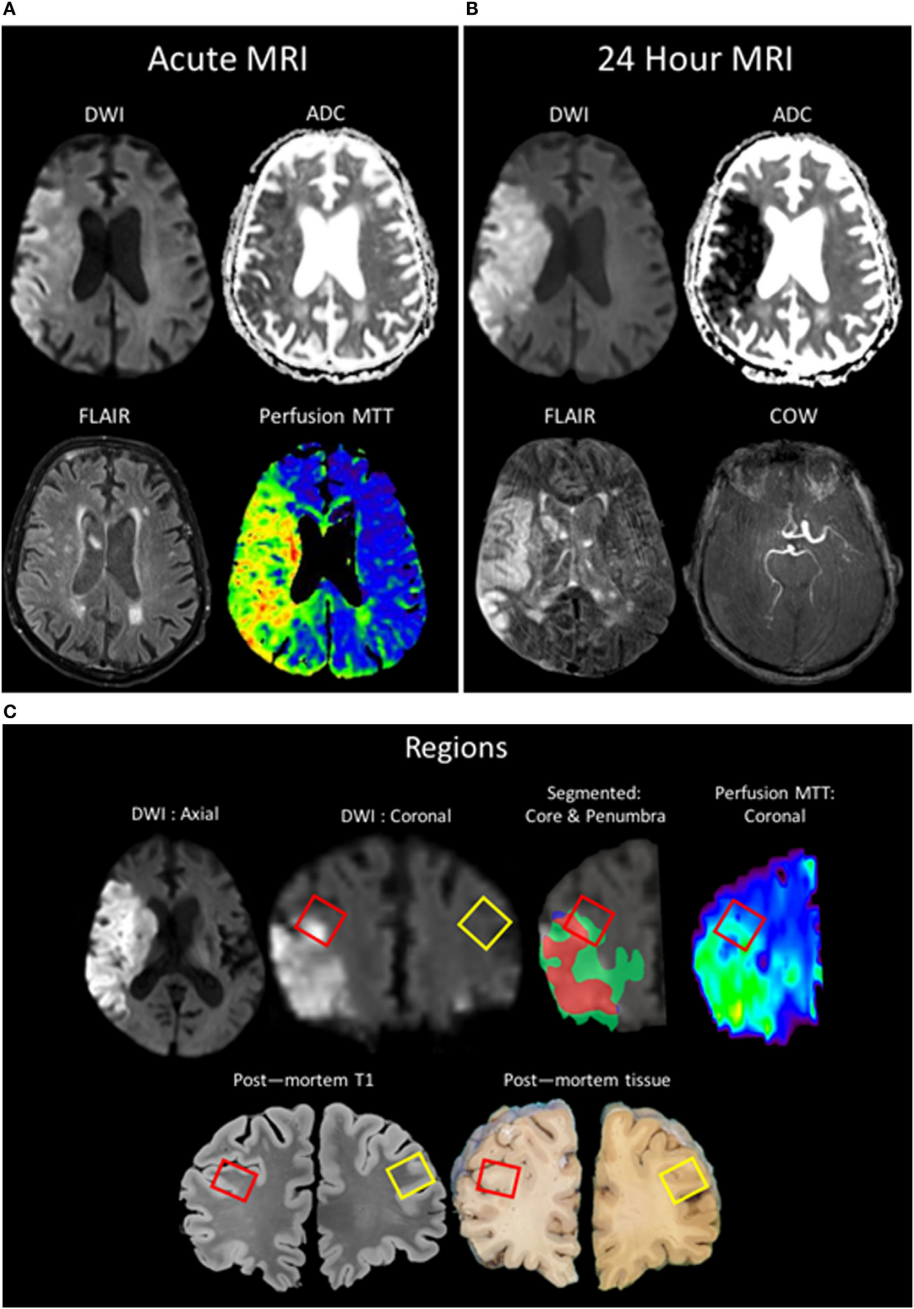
Patient MR images taken during clinical evaluation (acute) (A) and 24 h later (B) [pictured: trace-weighted diffusion weighted imaging (DWI) and ADC maps, fluid-attenuated inversion recovery (FLAIR), perfusion mean-transit time (MTT), and a minimum intensity projection of the circle of willis]. (C) MR images taken postmortem were analyzed to co-localize regions of interest identified *in vivo* with the postmortem sample (red boxes: ipsilateral ROI; yellow boxes: contralateral). Two regions of interest were segmented, the region of the core (red) taken from the baseline DWI and the region of the penumbra as the mismatch between the MTT and the DWI (green), and overlaid onto the 24-h DWI.
